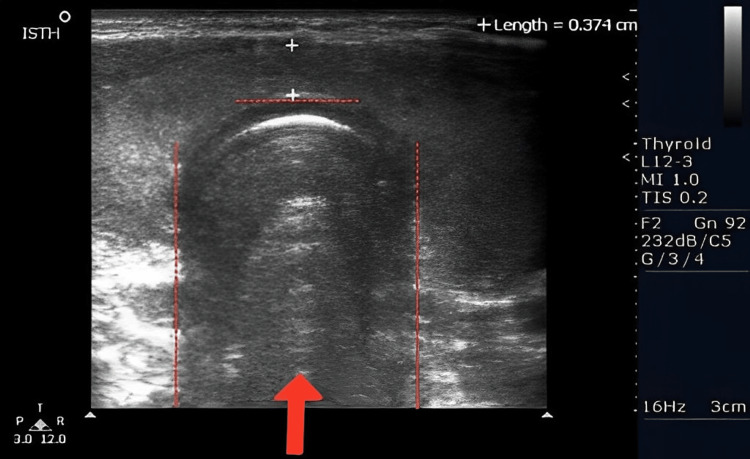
This figure depicts the whole isthmus. The red arrow and the region inside the dotted lines are the isthmus noted in the sonogram scan.
